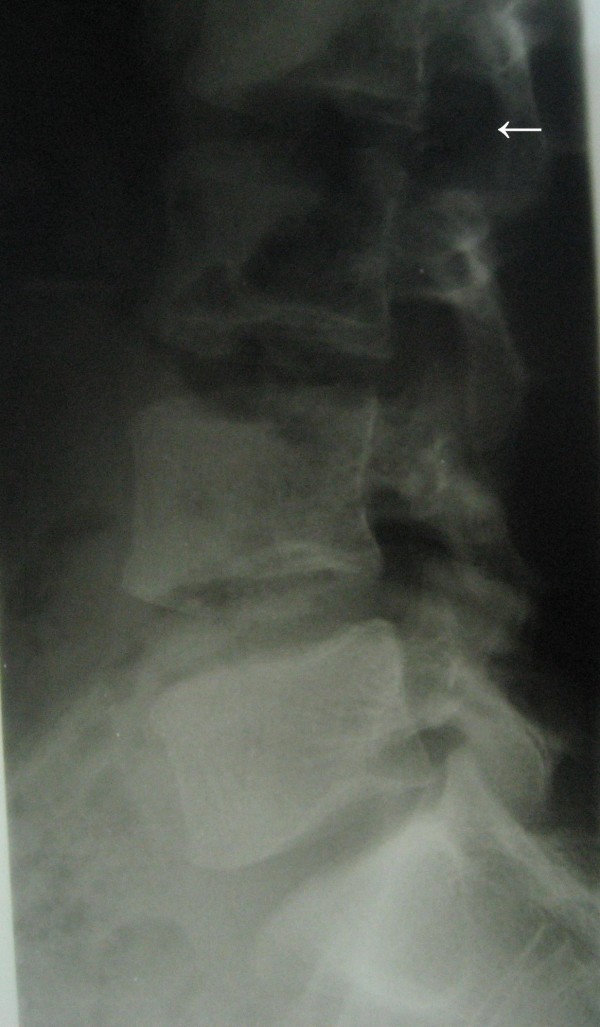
Plain radiography of lumbar area showing bony erosion and widening of the canal (scalloping) at the level of L3 vertebra (arrow).
